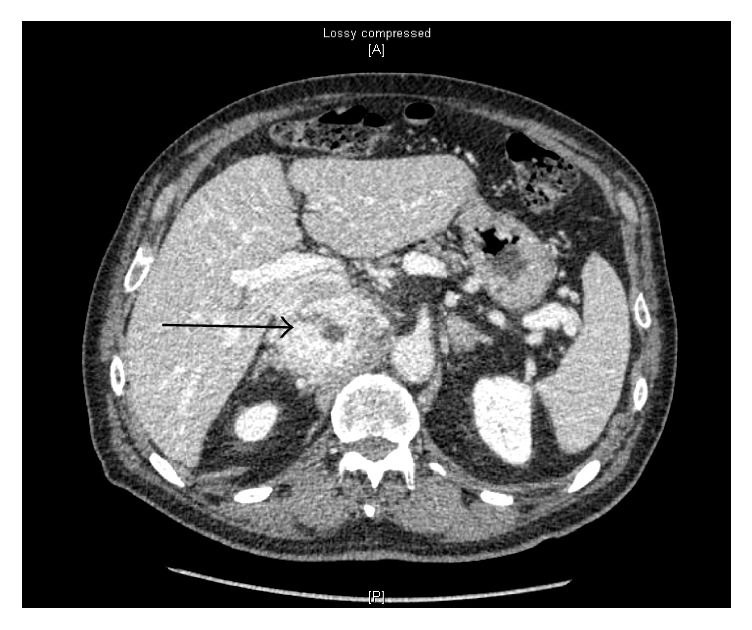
CT scan findings. 6.4 cm × 5.9 cm × 6.6 cm right retroperitoneal mass posterior to the retrohepatic inferior vena cava (arrow).
